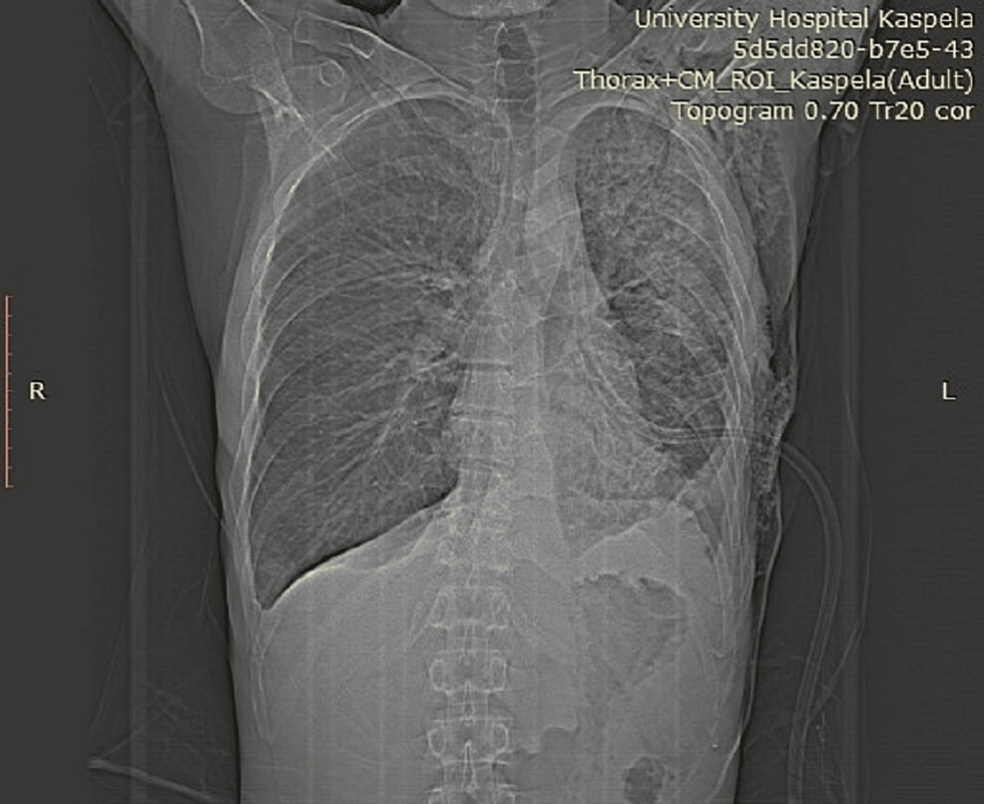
The CT just after a thoracostomy tube is placed, demonstrating a hydropneumothorax on the left
